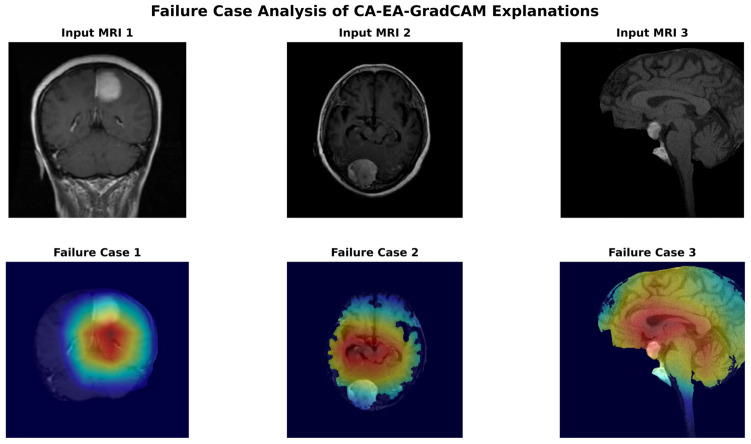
Failure case analysis showing representative MRI inputs and corresponding CA-EA-GradCAM outputs for samples with incorrect or uncertain predictions.
